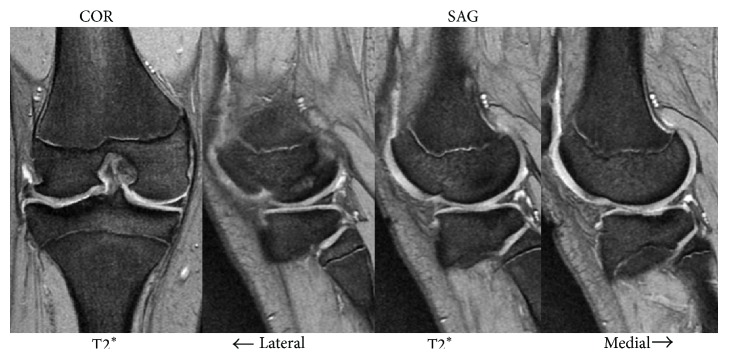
MRI showing that the defect of the tibial lateral condyle articular cartilage was covered with cartilage.
